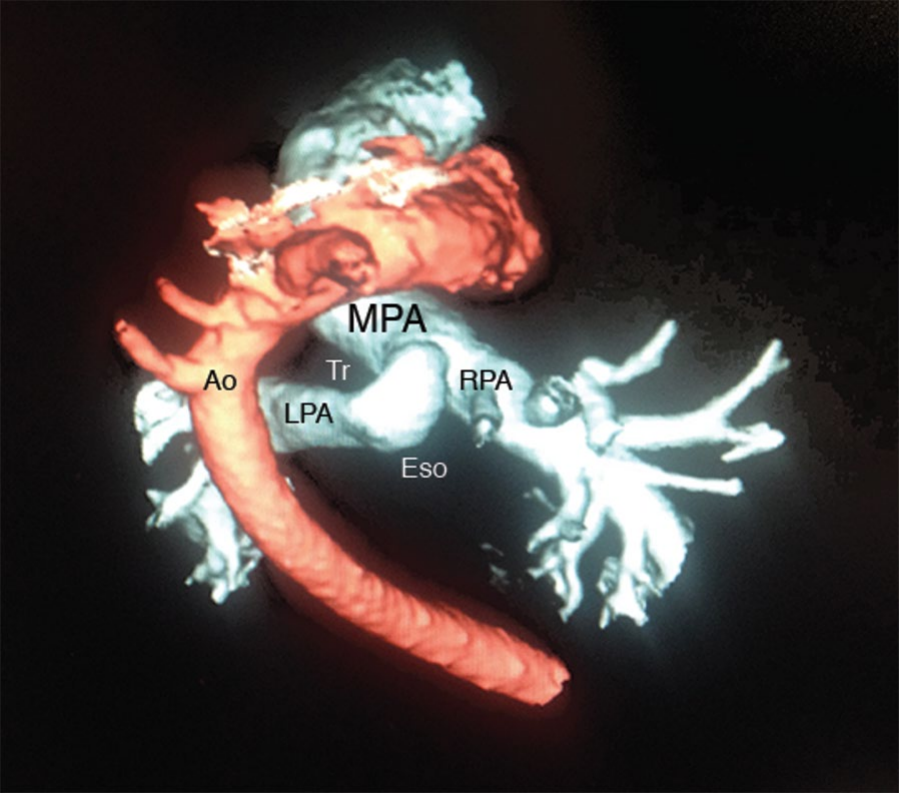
Posterior view of 3D computed tomography angiogram reconstruction showing the main pulmonary artery (*MPA*) giving rise to the left (*LPA*) and right (*RPA*) pulmonary arteries. A pulmonary artery sling is formed as the LPA makes an acute angle encircling the trachea (*Tr*), runs up against the esophagus (*Eso*), and finally passes anterior to the aorta (*Ao*)
